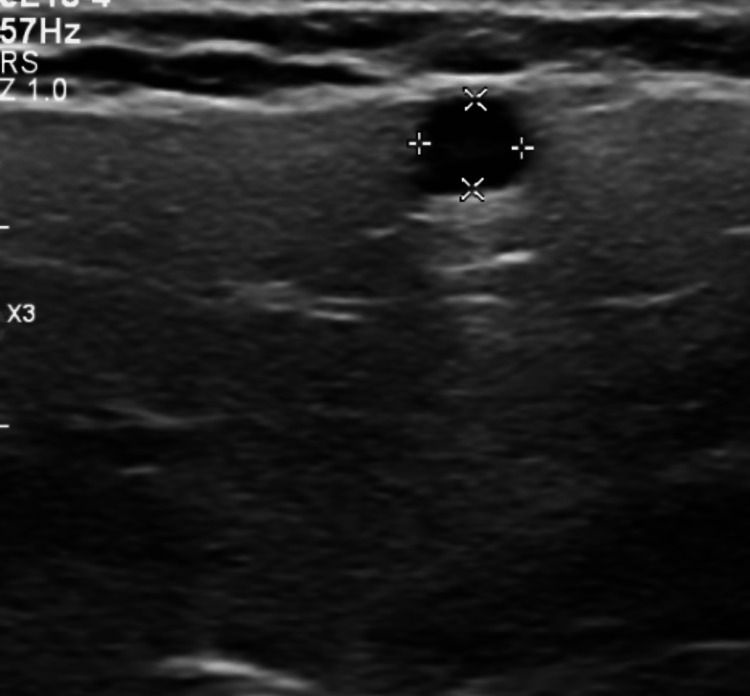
Ultrasound of the parotid gland showing the left parotid cyst.
